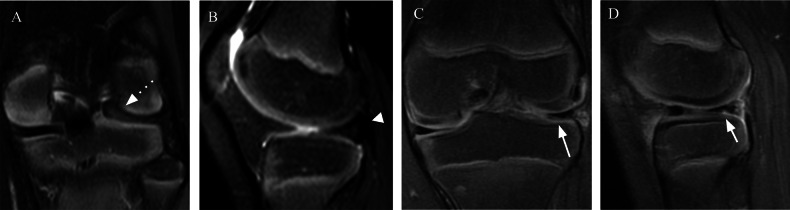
A 7-year-old female with a history of knee pain after trauma and a postsurgical evaluation 3 months later. A, B Coronal and sagittal T2FS: presurgical MR image showing a posteriorly subluxated DM (arrowhead) due to a horizontal tear (dotted arrow) with separation of the anterior meniscocapsular junction (not shown) and a posterocentral shift. C, D Coronal and sagittal T2FS: meniscus image 3 months after surgery showing a volumetric reduction, contour irregularities, and shortening/amputation of the free margins and foci of the intrasubstantial signal changes related to previous manipulation (arrows)
